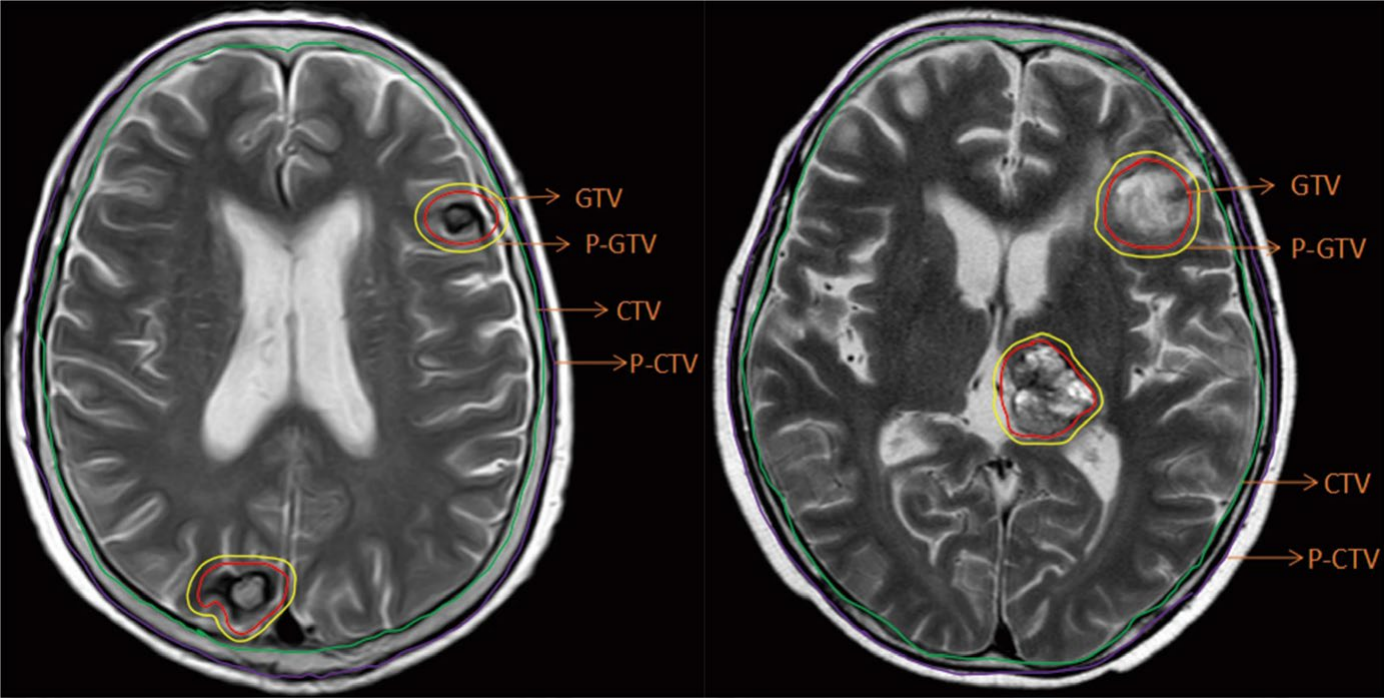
Delineation of important RT volumes (GTV, CTV, PTV, etc) on T2‐weighted MRI. CTV, clinical target volume; GTV, gross tumor volume; MRI, magnetic resonance imaging; PTV, planning target volume
